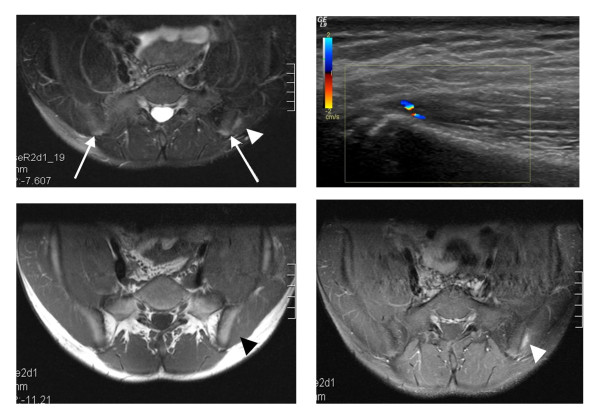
US and MRI images of a 15 year old boy with JIA and enthesitis. A) Fat suppressed T2-weighted MRI sequence showing bilateral, physiological edema of the iliac crests (arrows). Focal muscle edema at the left *gluteus medius *insertion (arrowhead) indicates enthesopathy. B) Longitudinal US with CD of the left insertion showing a hypoechoic area and hyperemia. C) T1-weighted MRI sequence before intravenous gadolinium contrast injection. D) Fat suppressed T1 sequence after contrast injection showing enhancement (arrowhead) in the muscular insertion.
